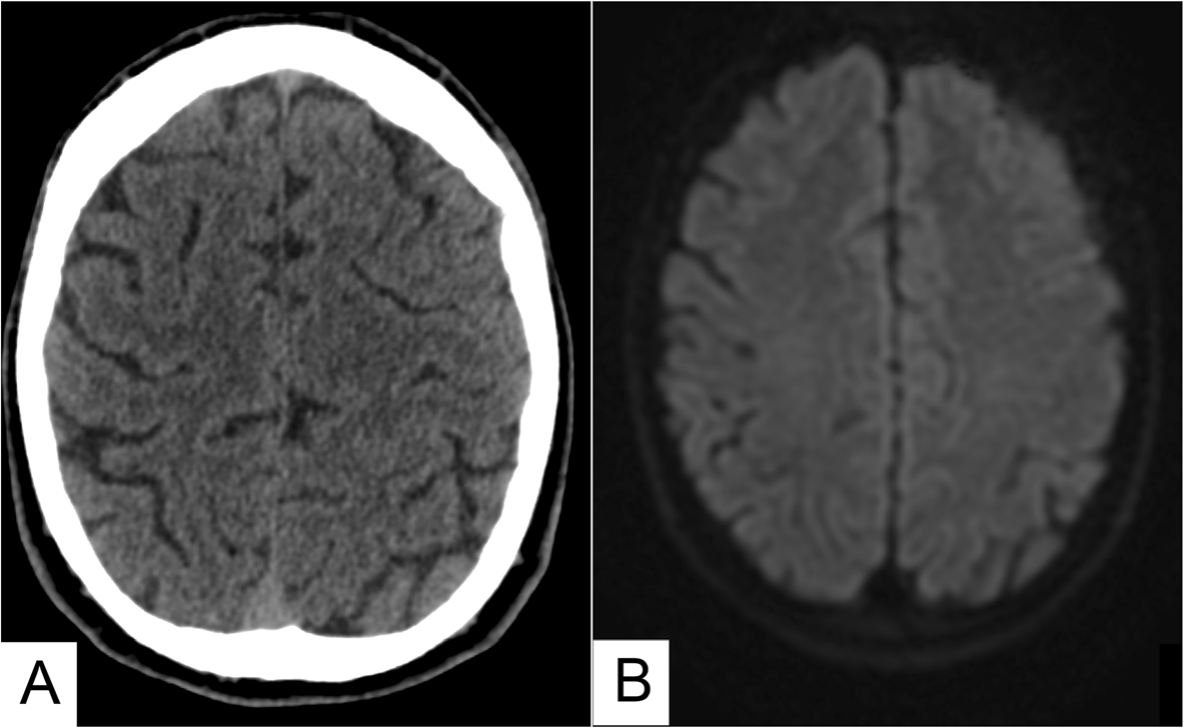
Nine months CT (a) and MRI (b) control. Complete disappearance of migrated material
